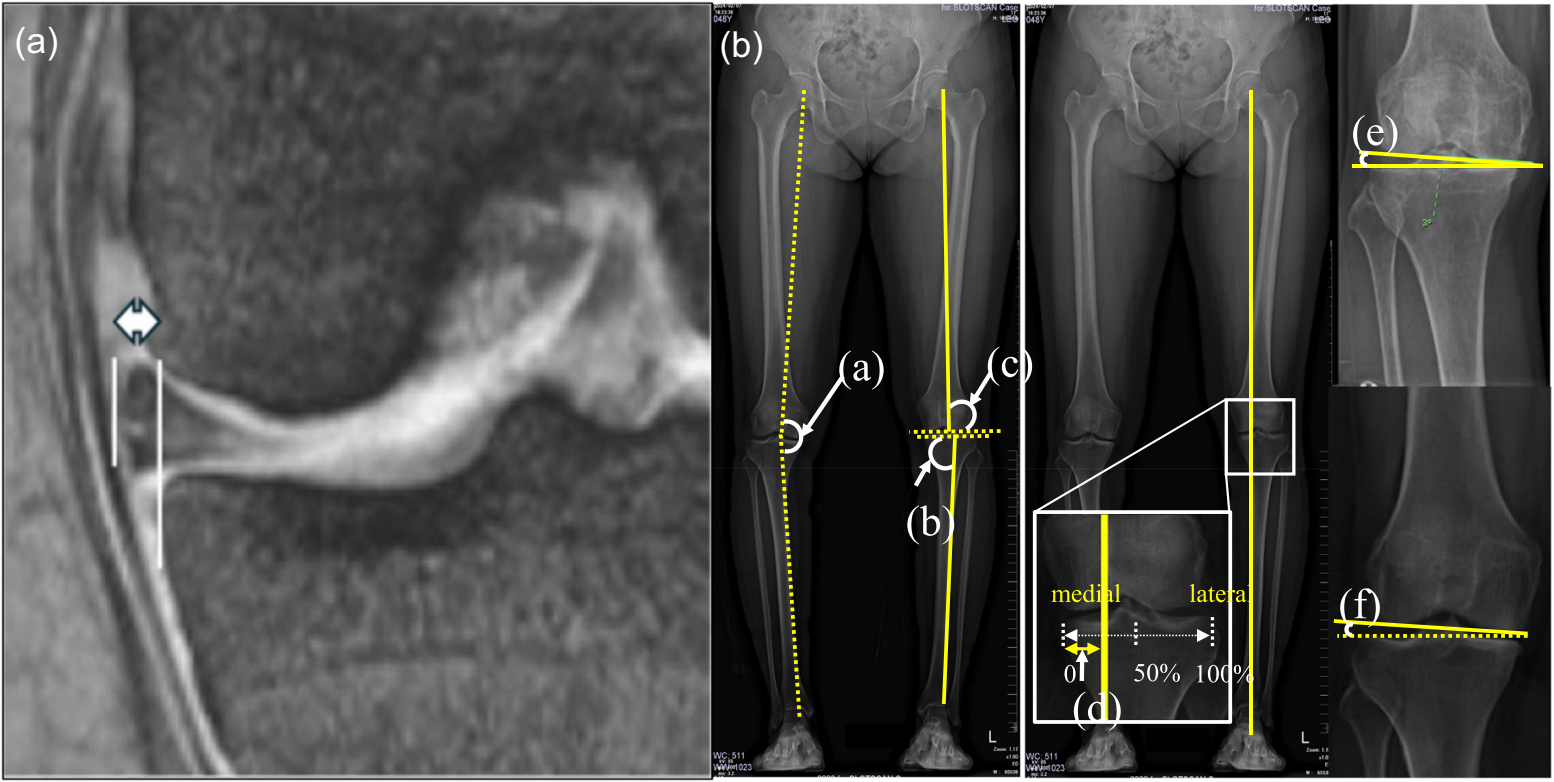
(A) Measurement of MME on the coronal MRI image. The measurement of meniscal extrusion was conducted by determining the distance from the edge of the extruded meniscus to a parallel line drawn perpendicularly from the medial tibial margin, excluding the bone spur. (B) Measurement of radiological parameters on a long‐leg radiograph. (a) Hip–knee–ankle angle (HKA). (b) Mechanical medial proximal tibial angle (mMPTA). (c) Mechanical lateral distal femoral angle (mLDFA). (d) Percentage of mechanical axis (%MA). (e) Joint line convergence angle (JLCA). (f) Joint line obliquity angle (JLO). MME, medial meniscus extrusion; MRI, magnetic resonance imaging.
